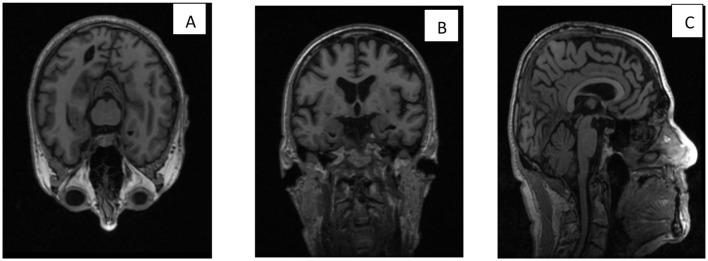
(A) Axial, (B) coronal, and (C) sagittal, views of brain MRI.
